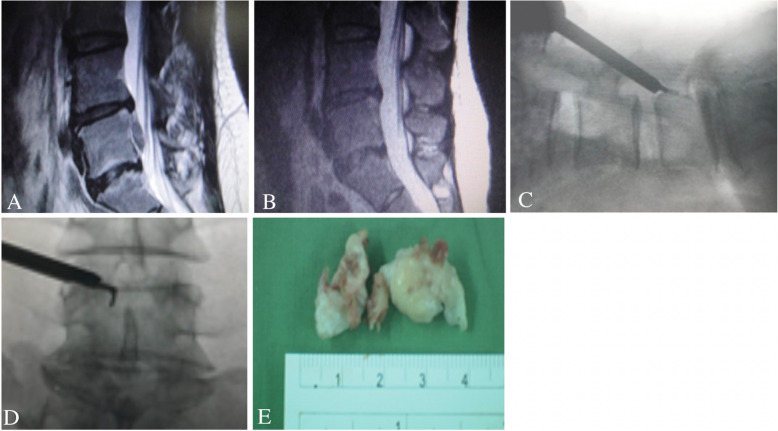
TELD for L4–L5 high-grade downward migrated herniated disc at zone 4. a Preoperative MRI lateral view showing an L4–L5 downward migrated disc. b Postoperative MRI lateral view showing complete decompression after the removal of the migrated disc. c and d Intraoperative fluoroscopy view showing flexible dissecting forceps probing down-migrated disc fragments at zone 4. e Removed disc fragments
